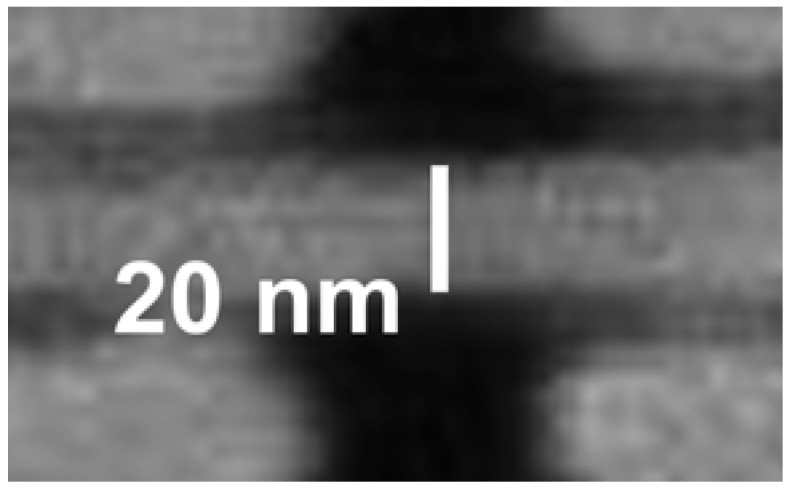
Magnified picture of a feature at the edge of the hard-X-ray FZPs of Figure 20 and Figure 21.
